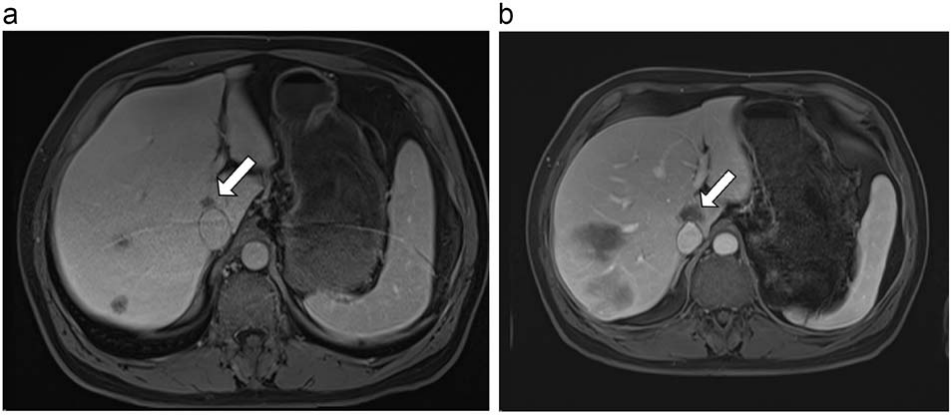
(a) Pre-ablation MRI demonstrating 14 mm lesion in caudate lobe of liver. (b) Post-ablation MRI (23 days postoperatively) demonstrating 23.8 mm complete ablation of caudate lesion.
